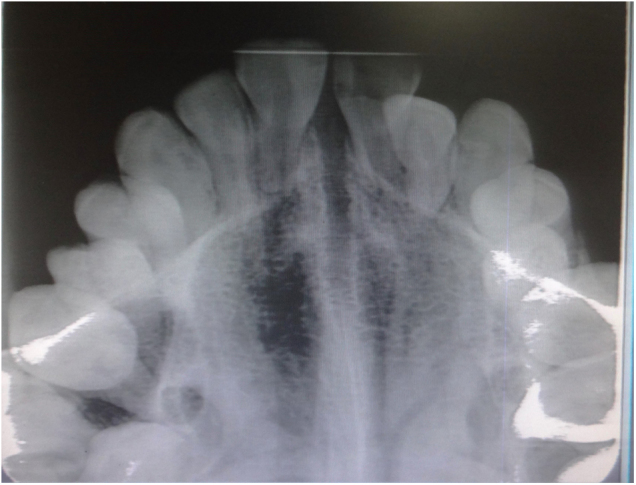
Occlusal view radiograph showing fan-shaped opening of the midpalatal suture in the same patient.
